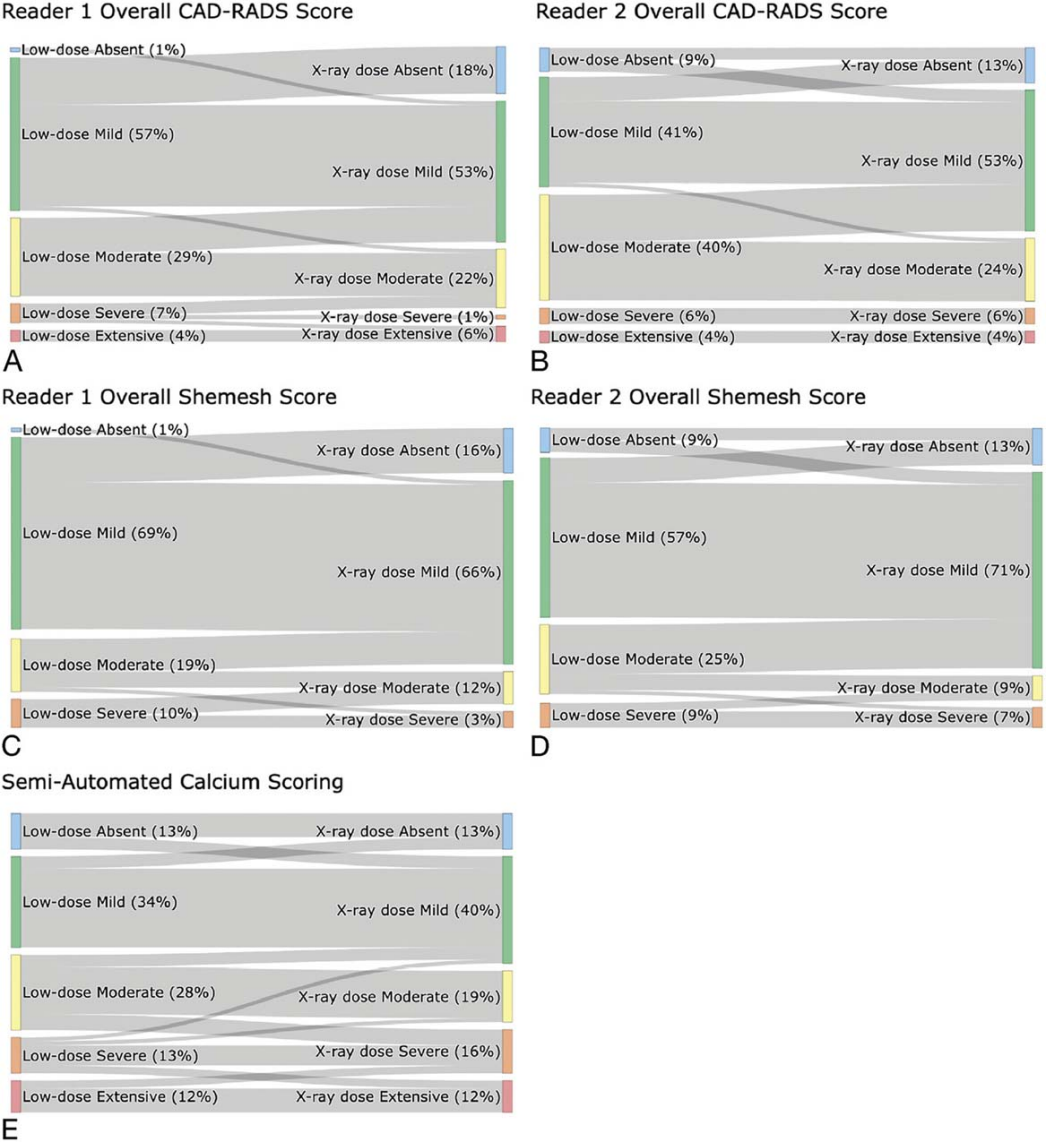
Sankey-Plots showing changes in classification from low-dose to radiography-dose CT for visual scoring using the CAD-RADS method for overall scoring (A and B) and the overall Shemesh score (C and D), as well as semiautomated analysis (E).
